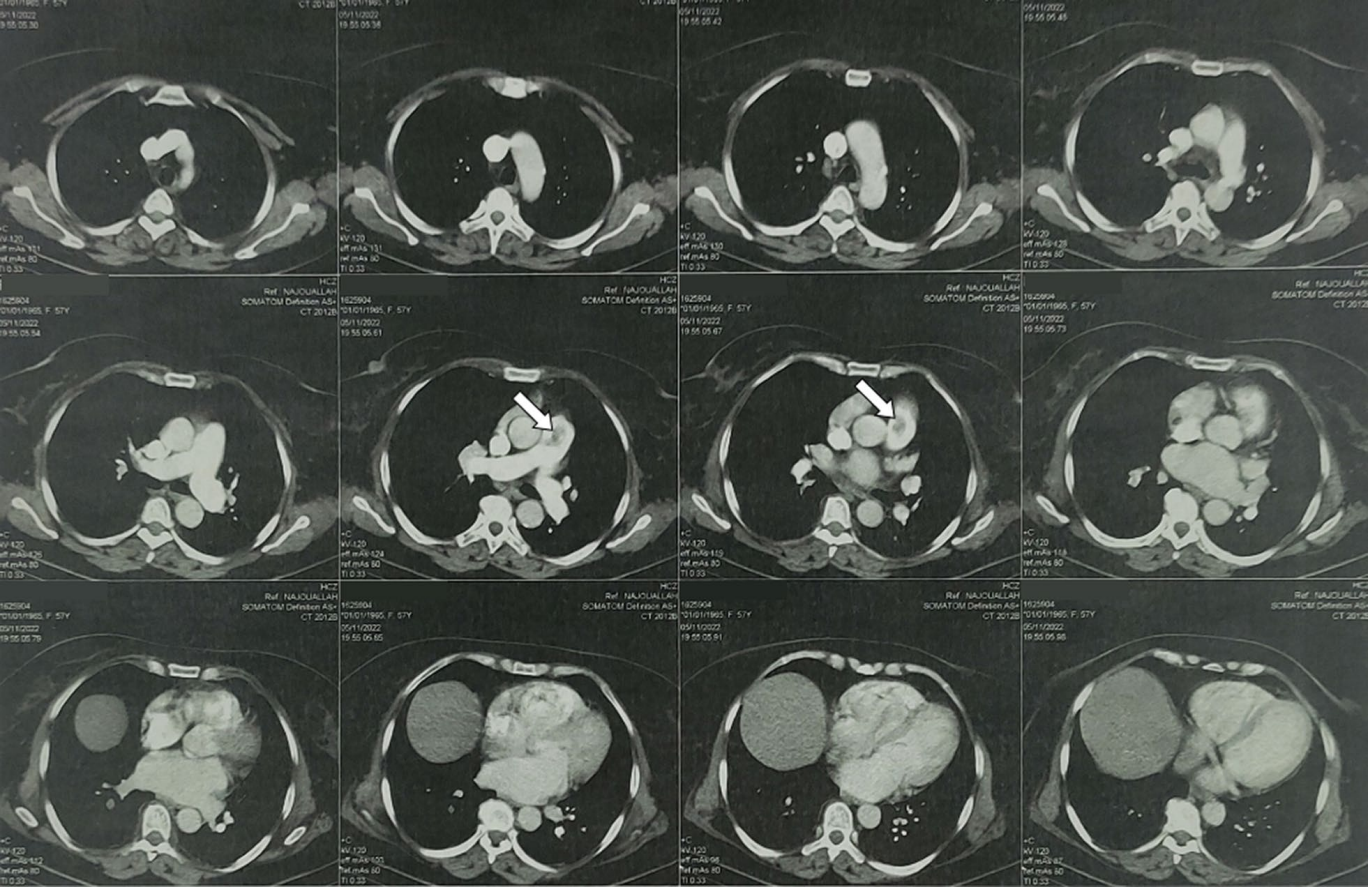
CT pulmonary angiogram showing proximal pulmonary embolism (white arrows)
